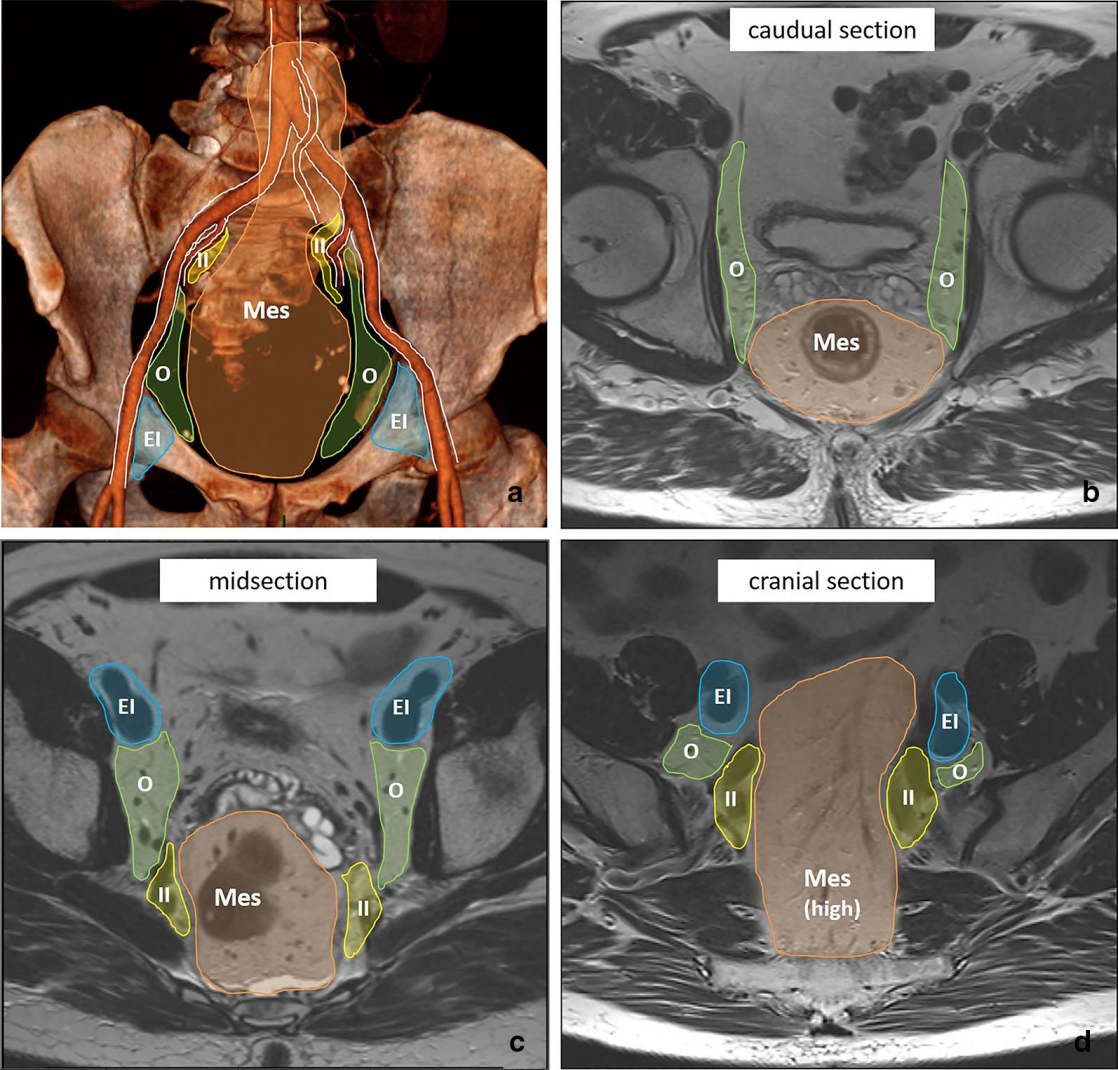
Overview of pelvic lymph node stations on MRI (a) with corresponding axial cross sections at the caudal (b), mid (c) and cranial (d) level. The mesorectal lymph nodes (Mes) in orange include all nodes in the mesorectal compartment, including the high mesorectal nodes that follow the superior rectal artery and vein towards the inferior mesenteric vein. The obturator nodes in green, are located dorsal from the external iliac vein and lateral from the lateral border of the main trunk of the internal iliac vessels, that separate the obturator (O) from the internal iliac (II) compartment in yellow. The external iliac nodes (EI) in blue are located alongside the external iliac vessels
